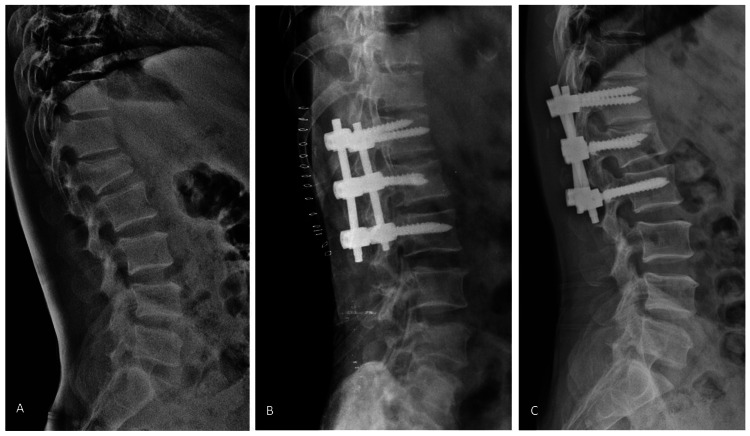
A 45-year-old male with AO type B2 L1 injury. (A) Preoperative radiograph. (B) Postoperative radiograph after short-segment fixation. (C) Radiographs at one year showing healing and intact fixation.
